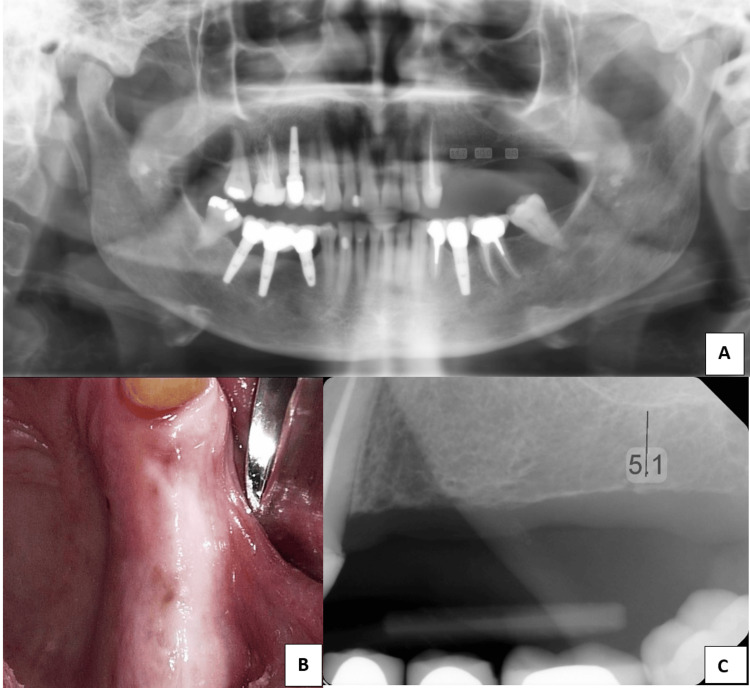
(A, B) Pre-surgical X-rays and clinical photograph. (C) The maxillary left ridge is severely resorbed, with noticeable pneumatization of the maxillary sinus.
